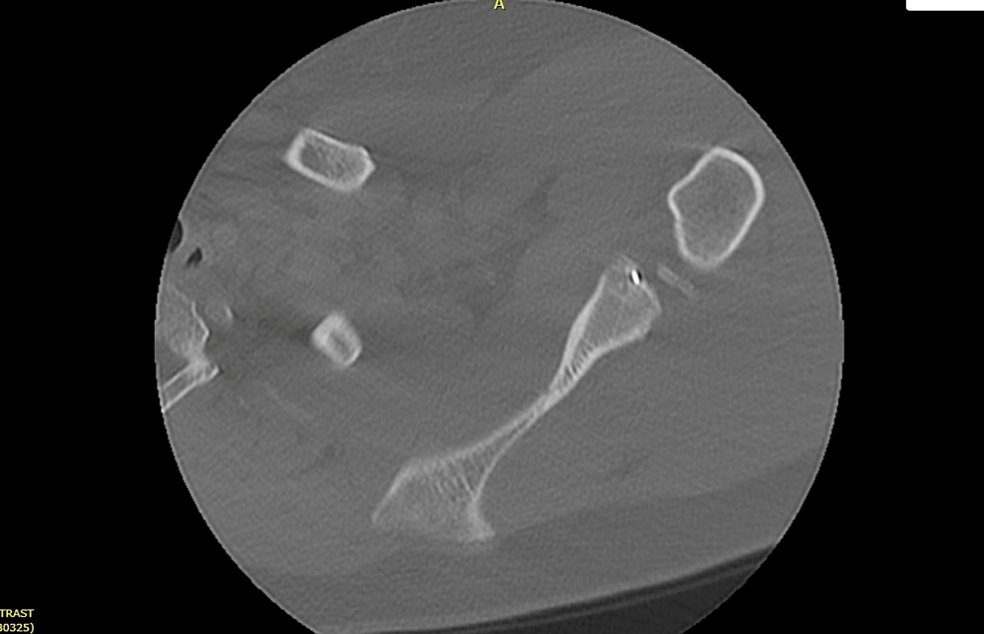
Axial cut CT image showing retained suture anchor at the anterior inferior glenoid rim.
